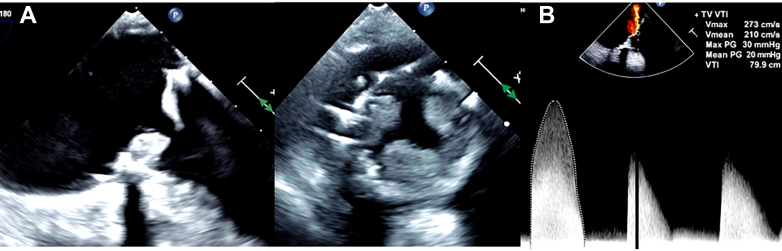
Intraoperative TEE (A) TEE midesophageal 4-chamber view showing severely thickened bioprosthetic tricuspid valves in the setting of thrombosis. (B) Continuous-wave Doppler across the tricuspid valve demonstrating severe obstruction before intervention (mean gradient: 20 mm Hg at a heart rate of 87 beats/min). TEE = transesophageal echocardiography.
